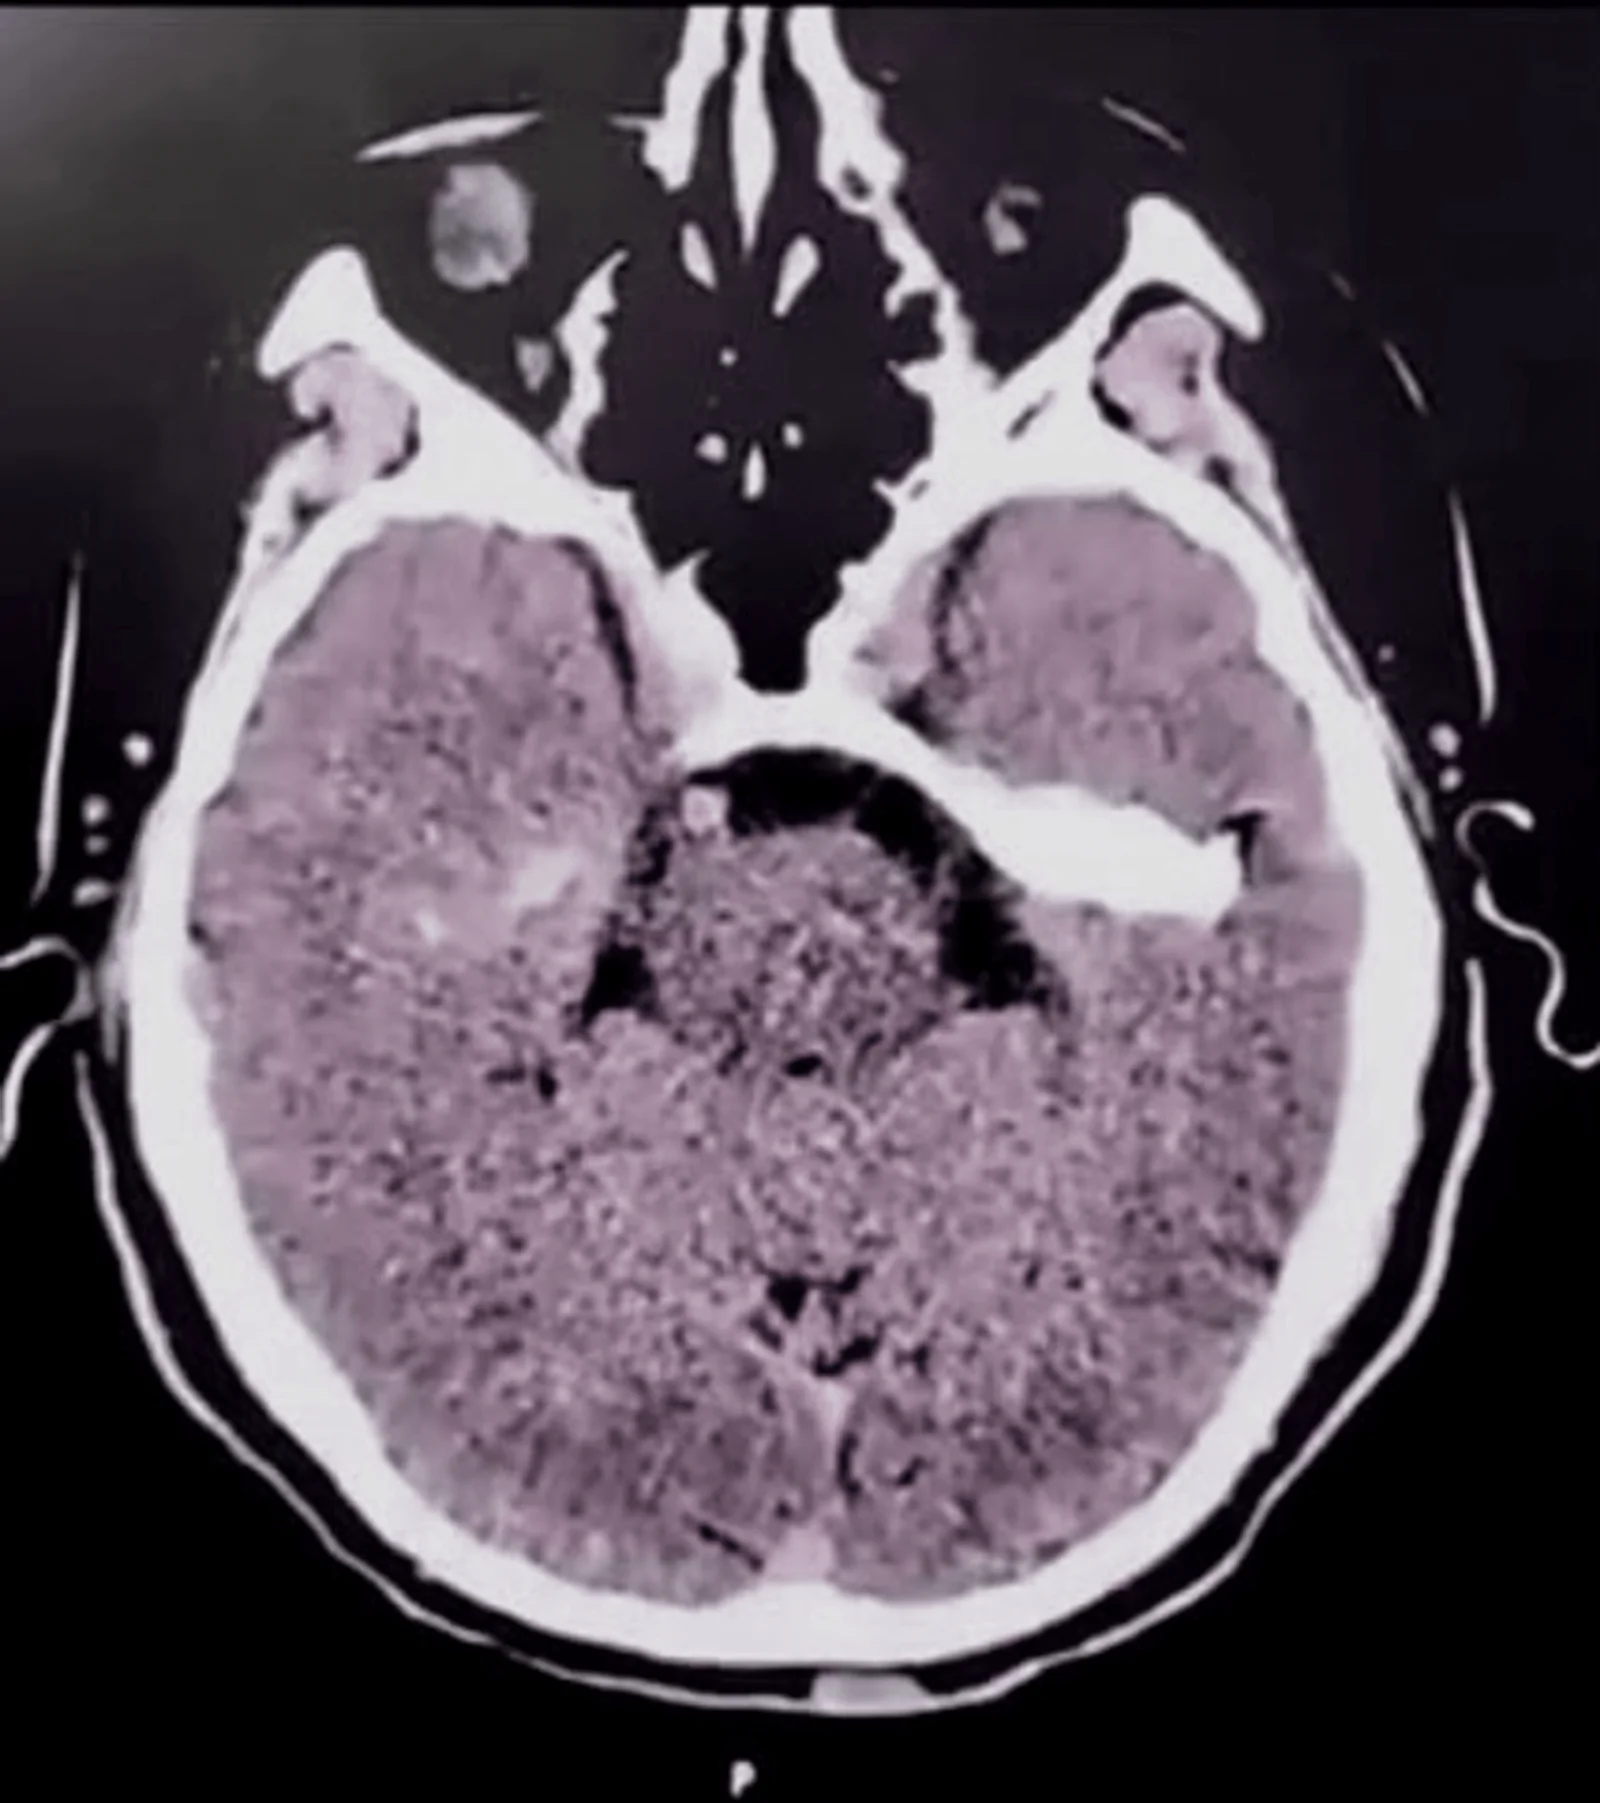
Axial non-contrast CT of the head at the level of the posterior fossa and basal cisterns.
